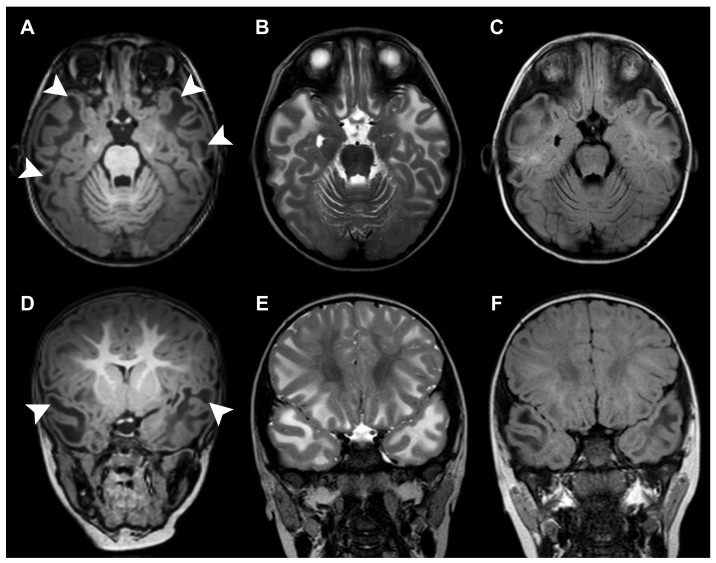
Brain magnetic resonance imaging (MRI) demonstrating a classic pattern of megalencephalic leukoencephalopathy with subcortical cysts (MLC). A 3-year-6-month-old girl with a history of febrile seizures and global developmental delay receiving rehabilitation was admitted because of a complex febrile seizure characterized by lateral gaze deviation and clonic movements lasting approximately 5 min. There was no family history of neurological or neurodevelopmental disorders. She had progressive macrocephaly since 18 months of age, with a head circumference of 53.7 cm (>97th percentile). Physical and neurological examinations revealed no focal deficits, and routine laboratory investigations were unremarkable. Electroencephalography revealed no epileptiform discharges. Brain MRI was performed. Axial (A–C) and coronal (D–F) brain MRI images are shown. T1-weighted images (A,D) demonstrate multiple hypointense cystic lesions in the subcortical white matter of the bilateral anterior temporal regions (arrowheads). Corresponding T2-weighted images (B,E) reveal extensive hyperintensity of the supratentorial white matter with a characteristic swollen appearance, predominantly involving the subcortical regions. On T2-weighted fluid-attenuated inversion recovery (FLAIR) images (C,F), the cystic components show relative signal suppression with surrounding white-matter hyperintensity. The overall imaging appearance, characterized by extensive supratentorial white-matter involvement with subcortical cysts most prominent in the anterior temporal lobes, is highly characteristic of MLC [1].
